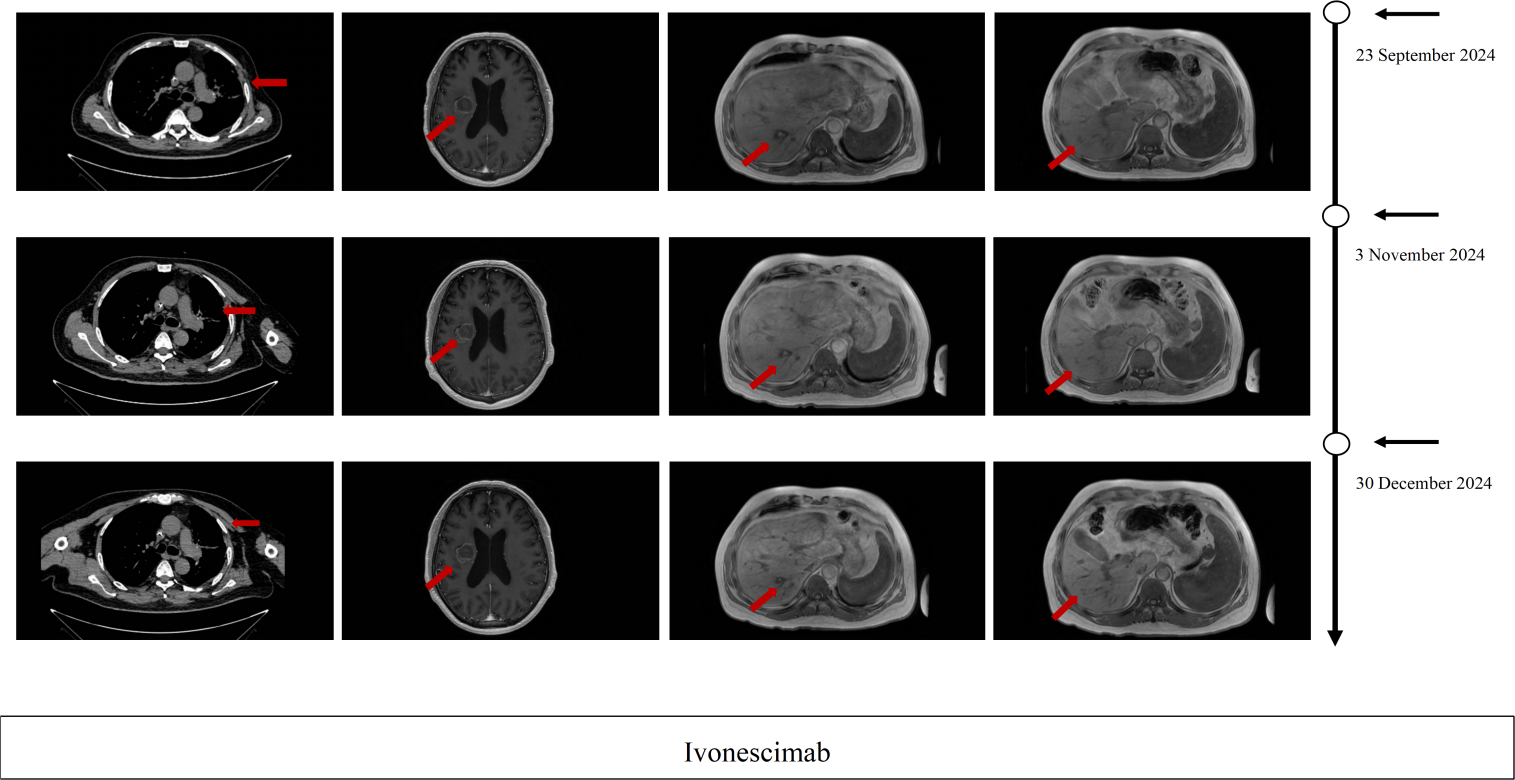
Imaging evaluation during ivonescimab. Therapy Serial thoracic CT, brain MRI, and hepatic MRI after 2 cycles, after 4 cycles and after 6cycles of ivonescimab therapy PR per RECIST 1.1 criteria.
